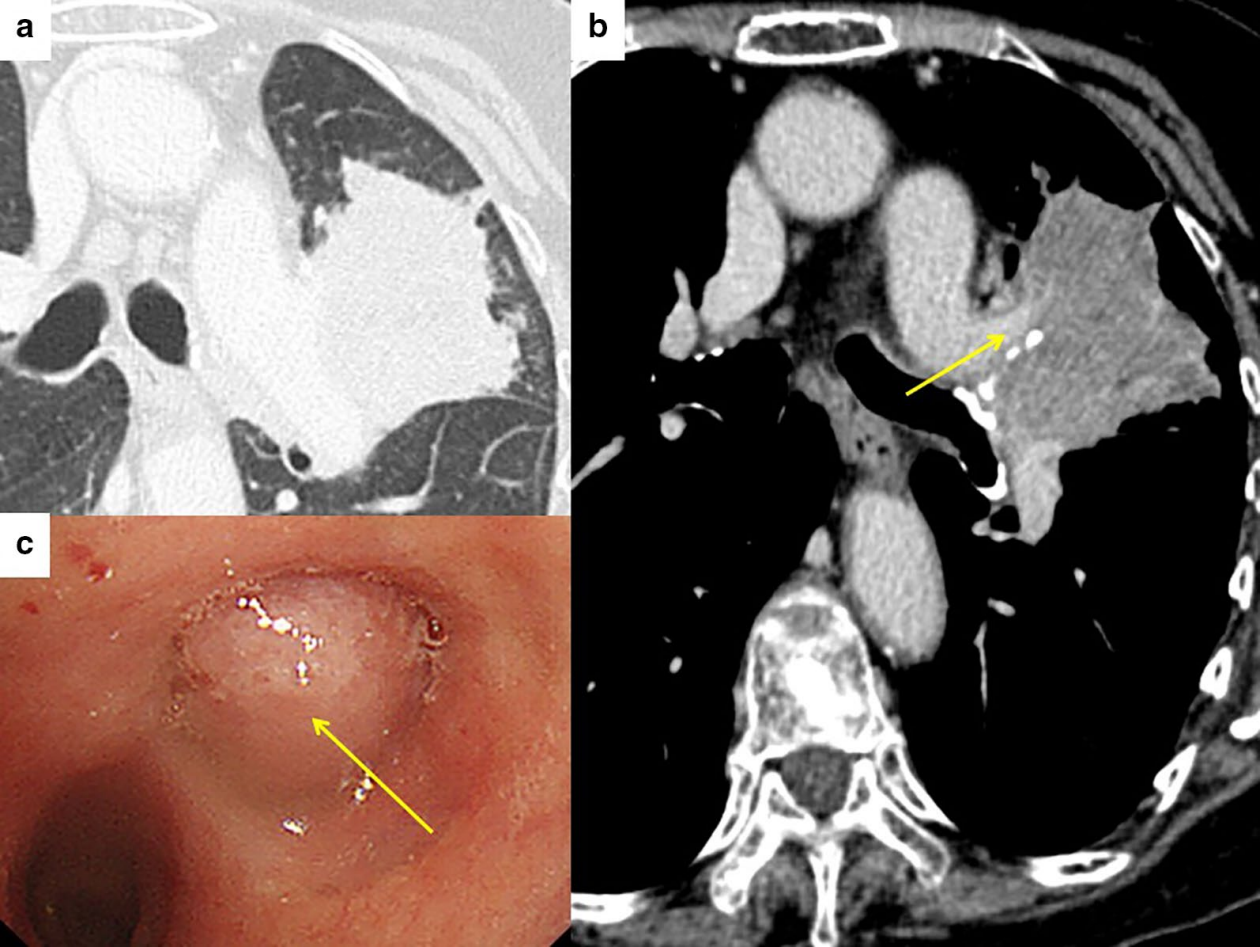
Pre-treatment enhanced chest computed tomography showed a solid mass measuring 6.2 cm in maximal diameter in the left-upper lobe (a) accompanied by narrowing of the left-upper lobe pulmonary artery surrounded by the mass (b). Bronchoscopy findings showed that the left-upper bronchus was completely obstructed by the polypoid tumor (c)
